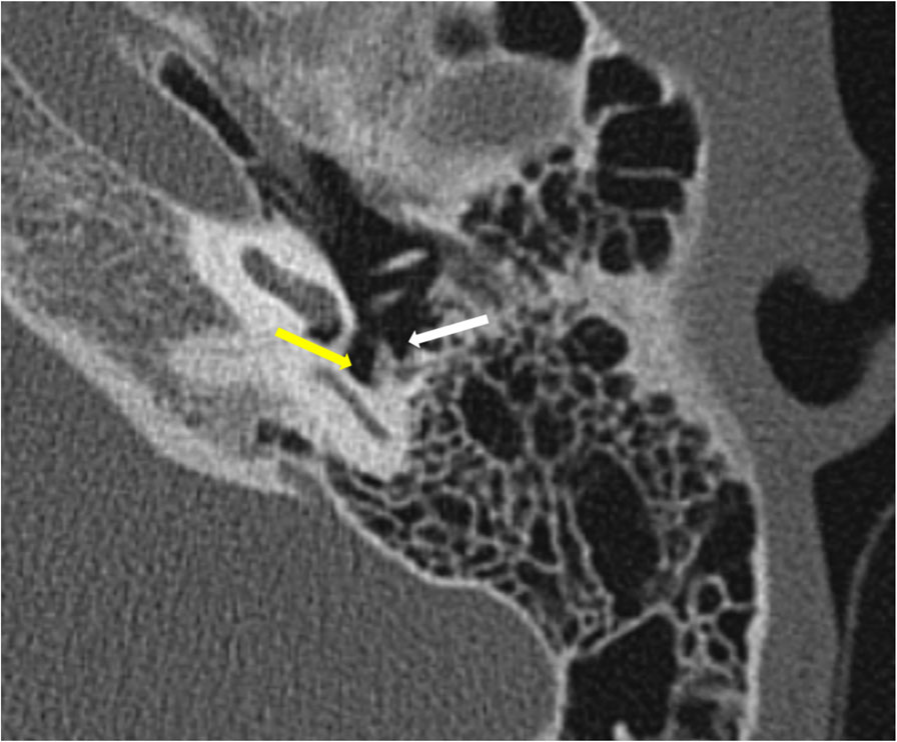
Axial CT through the mesotympanum shows the sinus tympani (yellow arrow) and facial recess (white arrow) which are hidden areas by otological examination and should be carefully inspected on imaging for the presence of soft tissue.
